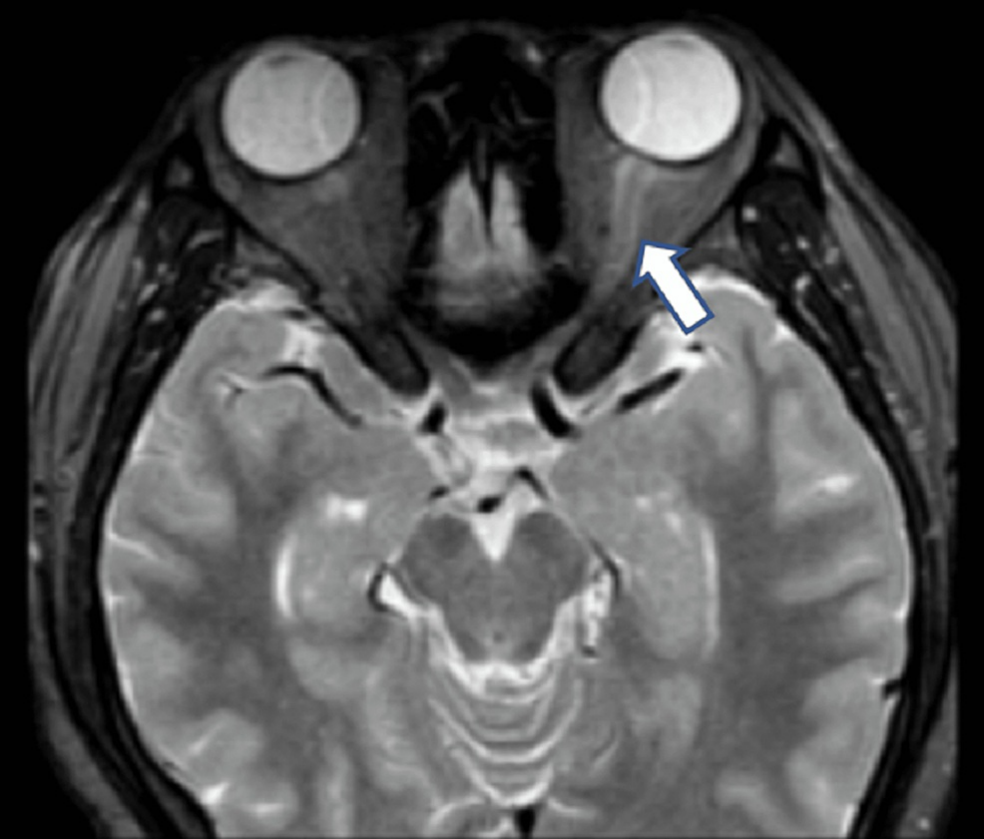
MRI of the orbit. Axial T2-weighted with gadolinium contrast showed enhancement (arrow) left optic nerve.
